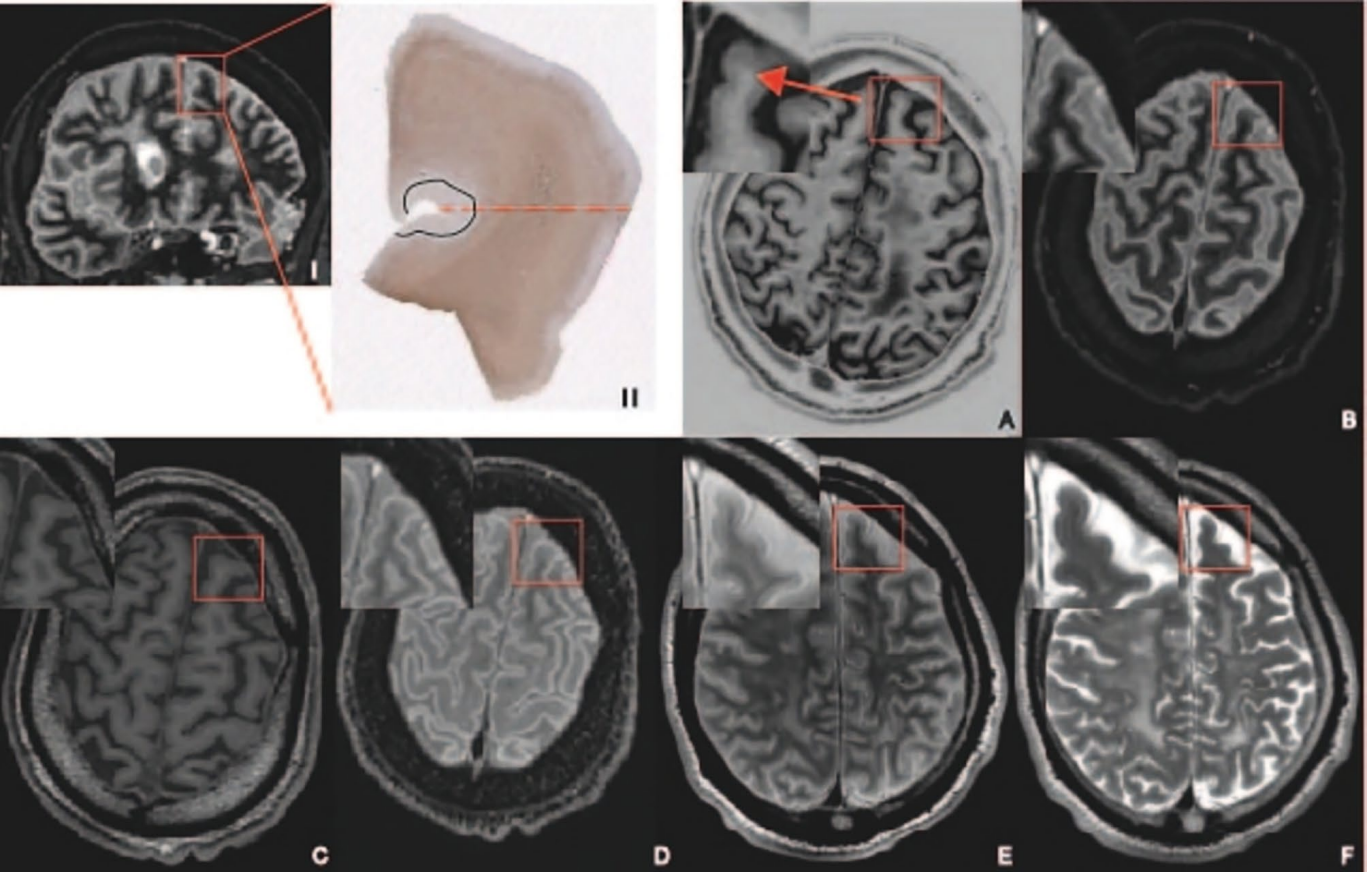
Example of a cortical lesion that is visible in the tissue but not on the MRI. (I) Coronal double inversion recovery image with red inset of tissue sample. (II) Tissue sample with a type III lesion demarcated by the black line. The red dotted line indicates the level of the axial MRI images in the tissue sample. A Phase-sensitive inversion recovery. B Double inversion recovery. C 3D-T_1_ weighted. D Fluid-attenuated inversion recovery. E Proton density. F T_2_ weighted
